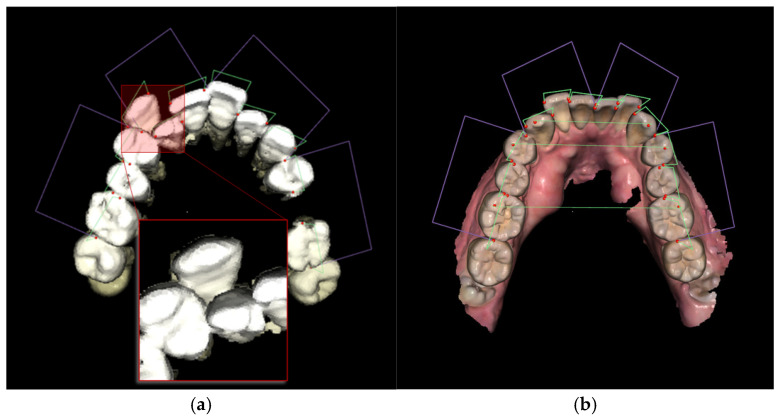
Example for solved abnormal test cases: (a) Abnormal teeth arrangement based on CBCT image; (b) abnormal teeth arrangement based on the scan image of the plaster dental model.
